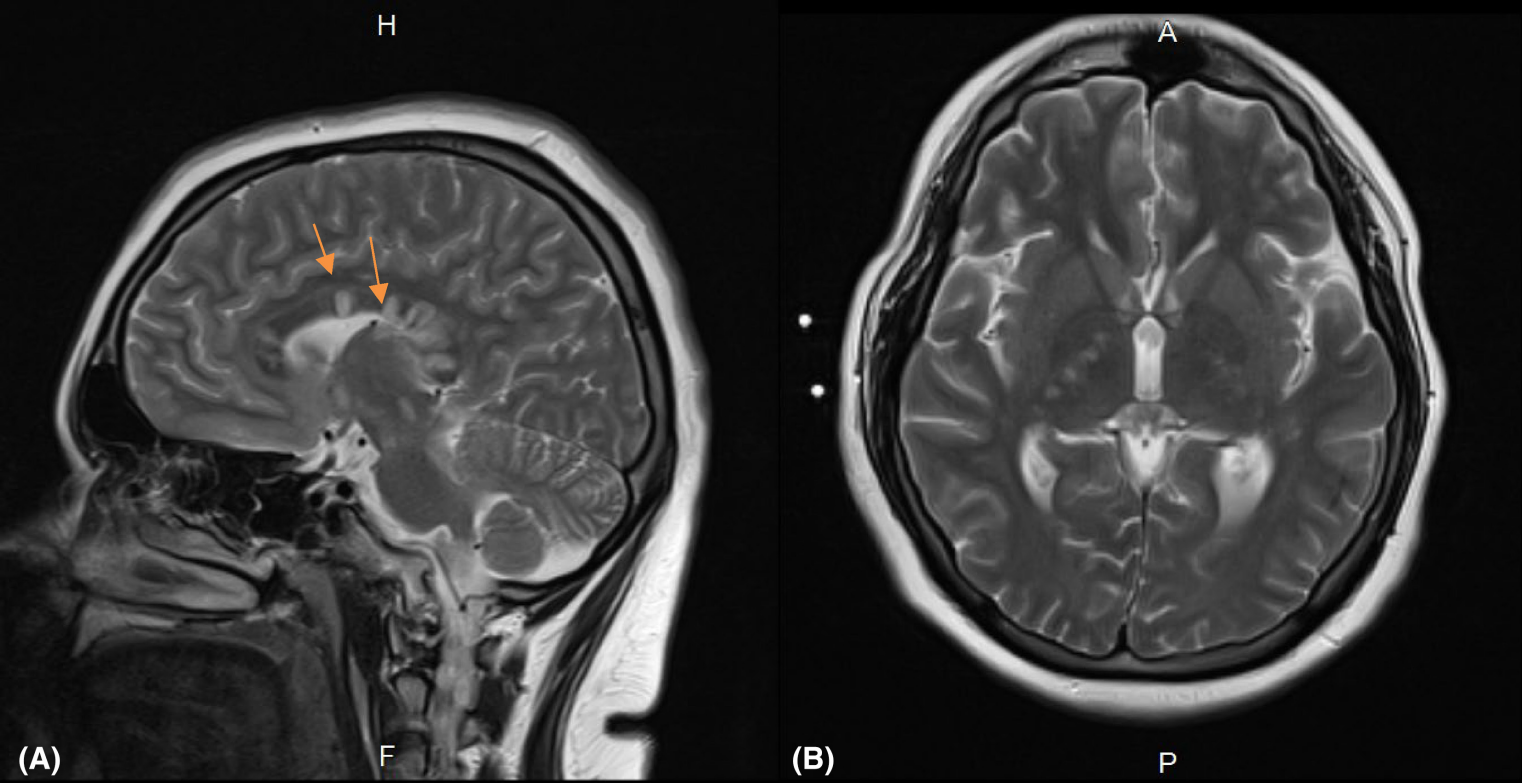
The patient's brain MRI demonstrating (A) hyper‐intense areas on T2‐weighted (yellow arrow), corpus callosum, there was evidence of snowballs, and periventricular and bilateral internal capsule, suggesting a string of pearls (B).
